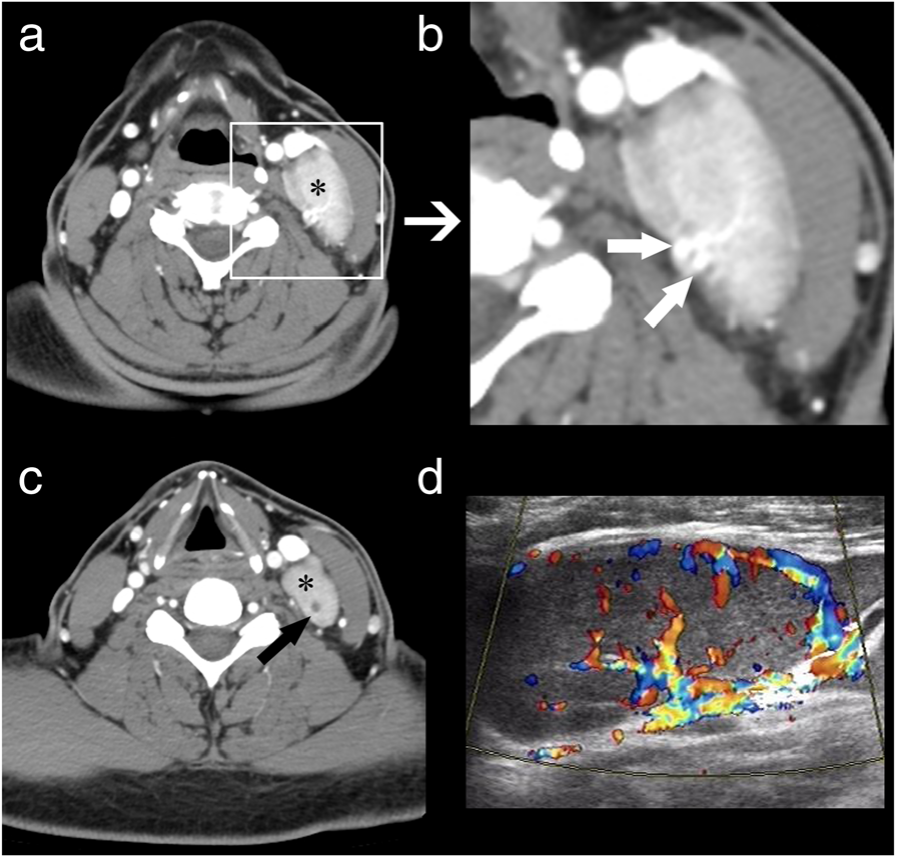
A 44-year-old man who presented with hyaline vascular type Castleman disease. a, c Contrast-enhanced CT images of the neck show multiple nodules (black *) with marked enhancement patterns. b Dilated and tortuous vessels are observable around the nodules. c An intra-nodular cystic region is also observable (black arrow). d Spectral Doppler ultrasound imaging shows plentiful blood flow
